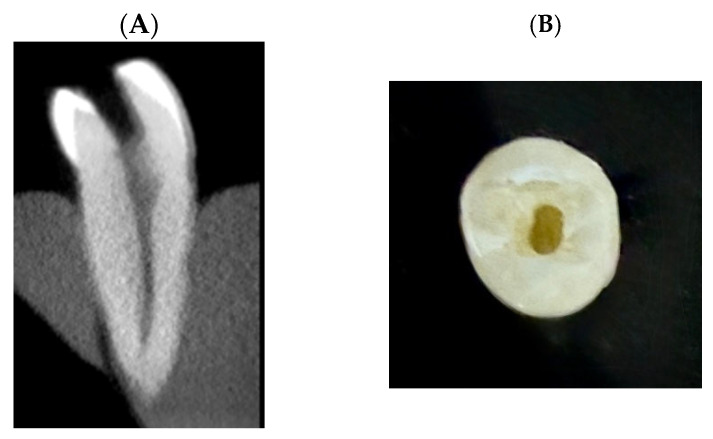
Representative sample showing the standardized access cavity preparation. All samples received a traditional endodontic access cavity to allow consistent canal instrumentation and irrigation. (A): CBCT Sagittal view of selected sample. (B): Occlusal photograph of the same sample showing the traditional access cavity.
